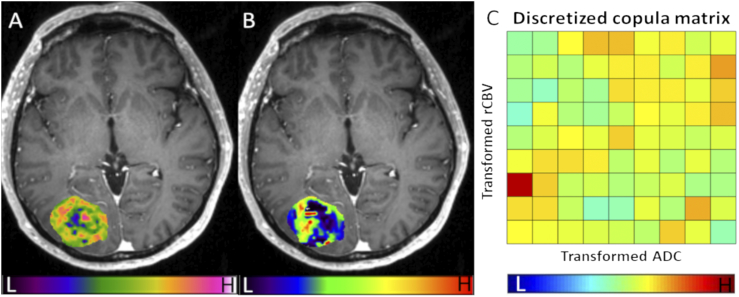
Case example of Subtype II. Pixel-wise ADC values (A) and rCBV values (B) are overlaid on postcontrast T1-weighted images. After the copula transform, the joint distribution is discretized (C). The matrix demonstrates a uniform distribution, which suggests a low interdependence of ADC and rCBV in this case.
